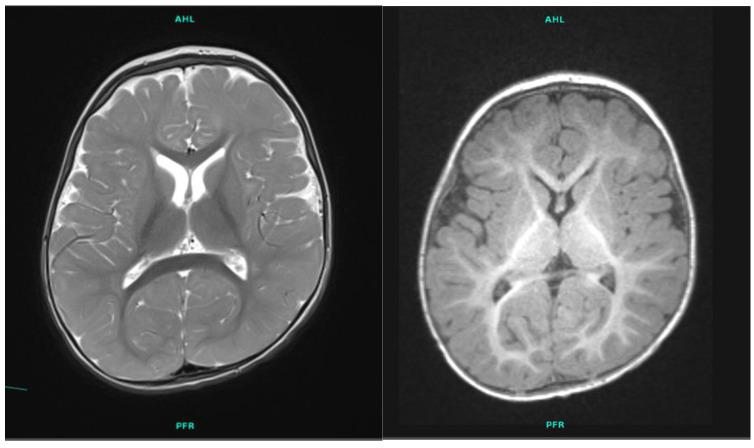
T2 and T1 weighted axial brain MRI images of patient 1 showing smaller basal ganglia and cerebral atrophy with fronto-temporal dominance.
